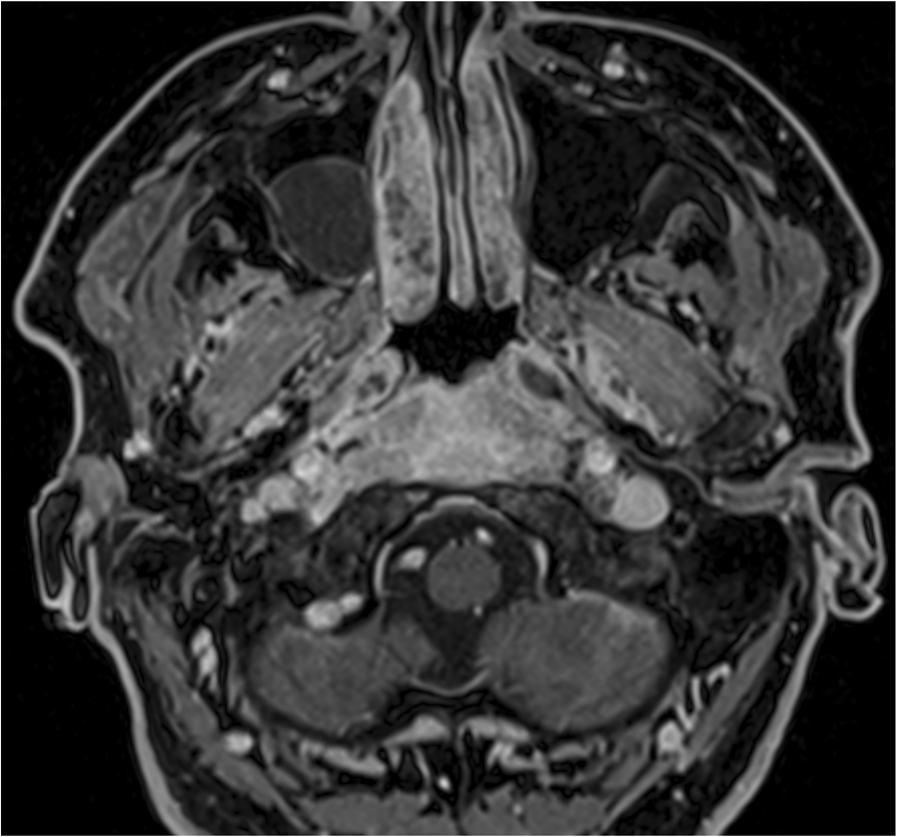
Day 209–477. Gadolinium-enhanced MRI, axial plane, T1-weighted, with fat suppression. Mild homogeneous signal increase persists in the pharyngobasilar fascia and longus colli muscles on both sides. A cyst in the right maxillary sinus represents a secondary finding
